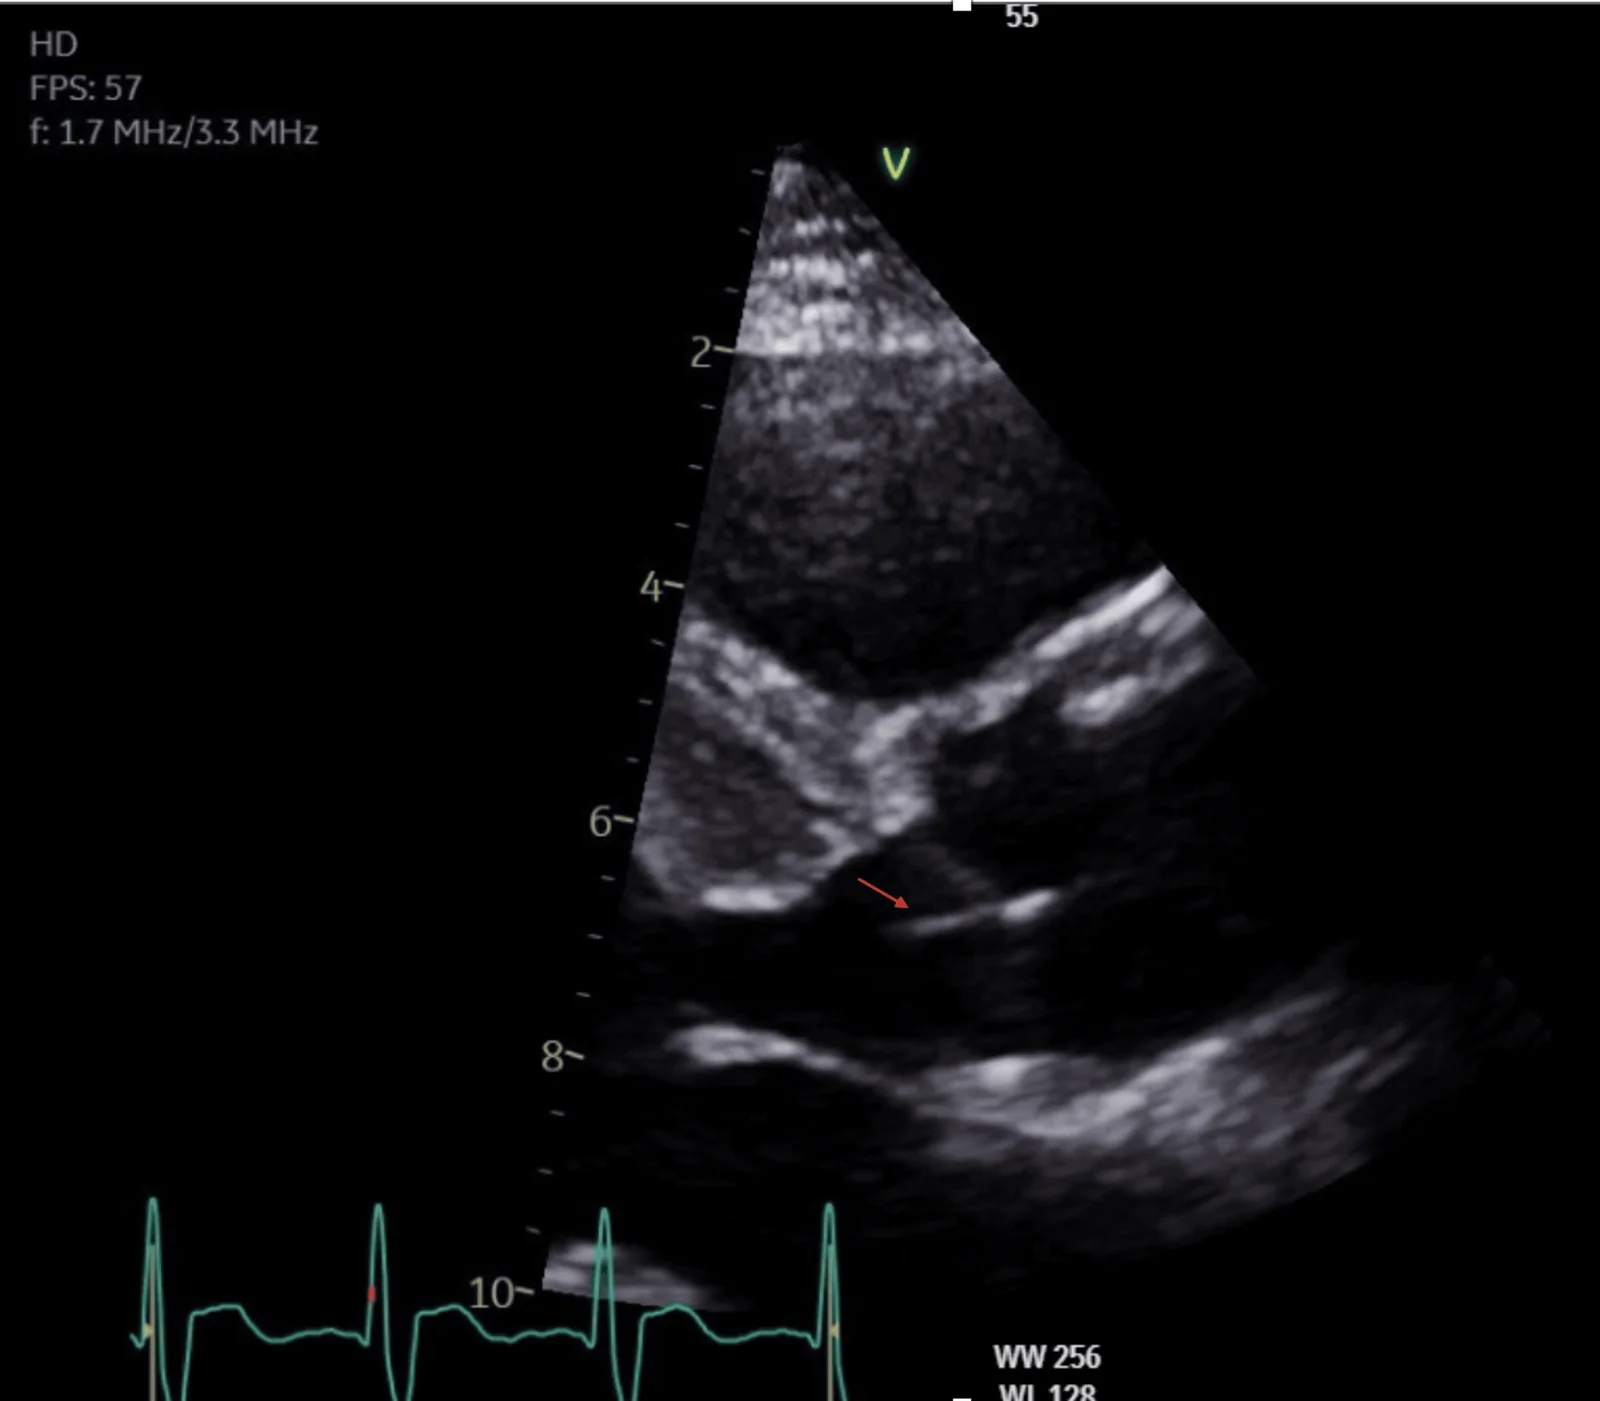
Transoesophageal echocardiography (TOE) findings: Focused parasternal long-axis view at the level of the aortic valve demonstrating a small, mobile, echogenic structure attached to the aortic valve The red arrow points to the small, mobile, echogenic structure attached to the aortic valve. This supported a diagnosis of endocarditis, although it was unclear whether this was infective or non-infective.
